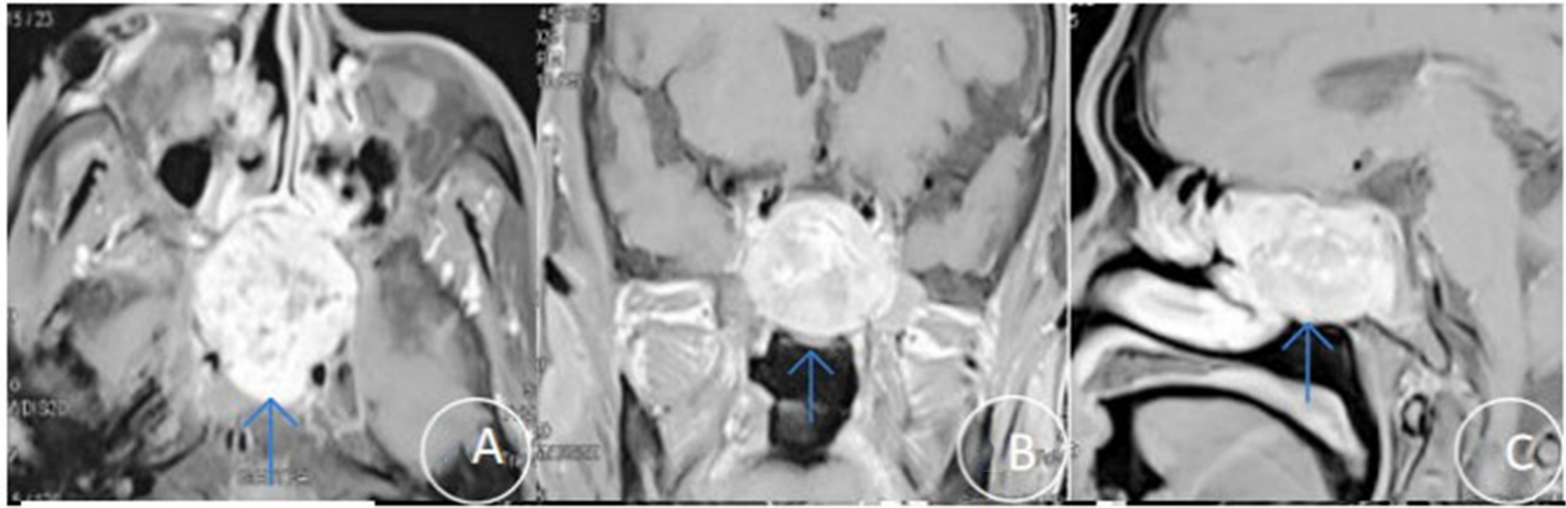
Preoperative MRI characteristics of a representative sphenoid sinus tumor. (A–C) Contrast-enhanced T1-weighted MRI (A) axial, (B) coronal, and (C) sagittal images delineate the tumor (arrows) invading the clivus, cavernous sinus, and protruding into the nasopharyngeal cavity.
